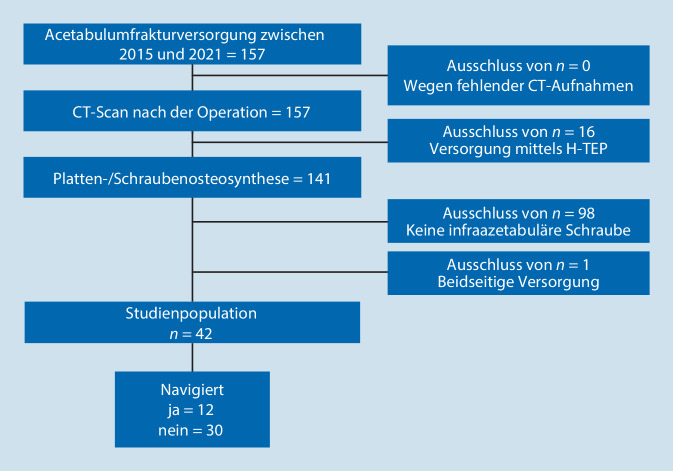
Die meisten Patienten wurden über einen modifizierten Stoppa-Zugang operiert. In 3 Fällen in der nichtnavigierten Gruppe war ein ilioinguinaler Zugang notwendig. Nach offener Reposition der Acetabulumfraktur wurde die Reposition mittels Fluoroskopie in a.-p.- und in Ala‑/Obturator-Aufnahmen kontrolliert (Abb. 2). In der navigierten Gruppe erfolgte zusätzlich eine 3D-Fluoroskopie mit digitaler Volumentomographie (Ziehm RFD 3D, Fa. Ziehm Imaging, Nürnberg, Deutschland) als Datengrundlage für die Navigation. Für die präoperative Planung und Navigation wurde das System der Fa. Brainlab (BrainLab curve, Fa. Brainlab, München, Deutschland) verwendet. Mit der chirurgischen Navigation kann der Bohrvorgang auf Basis der intraoperativen Bildgebung in Echtzeit kontrolliert werden. Über das optische Tracking des Bohrers verändert sich die multiplanare Reformation (ähnlich einer CT-Rekonstruktion) dynamisch, sodass der Bohrkanal präzise dargestellt wird. Das Eindrehen der Schraube erfolgt ohne zusätzliche Bildsteuerung. Der idealer Eintrittspunkt der IAS wurde von Culemann et al. beschrieben [9, 10]. Die übrigen Operationsschritte zur Frakturstabilisierung wurden in der Technik nach Letournel durchgeführt [11].
